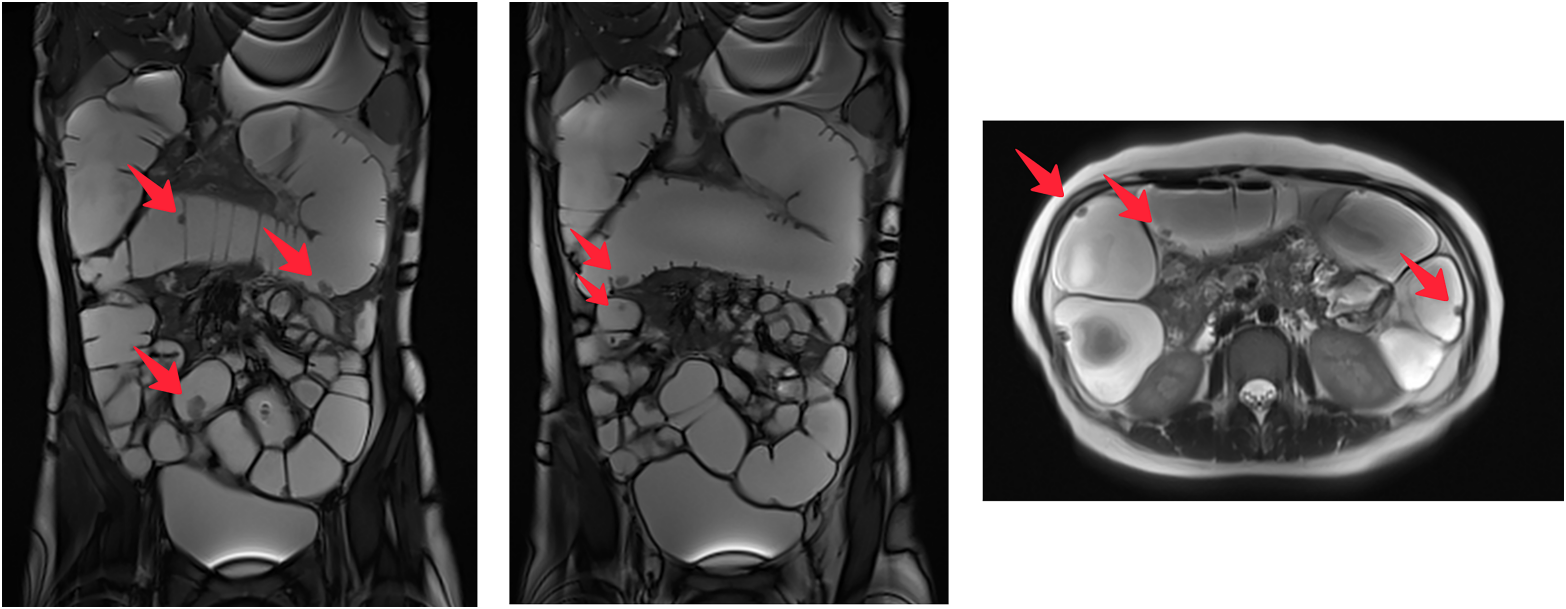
Magnetic resonance imaging showing abnormal signals at various sections of the intestine.
